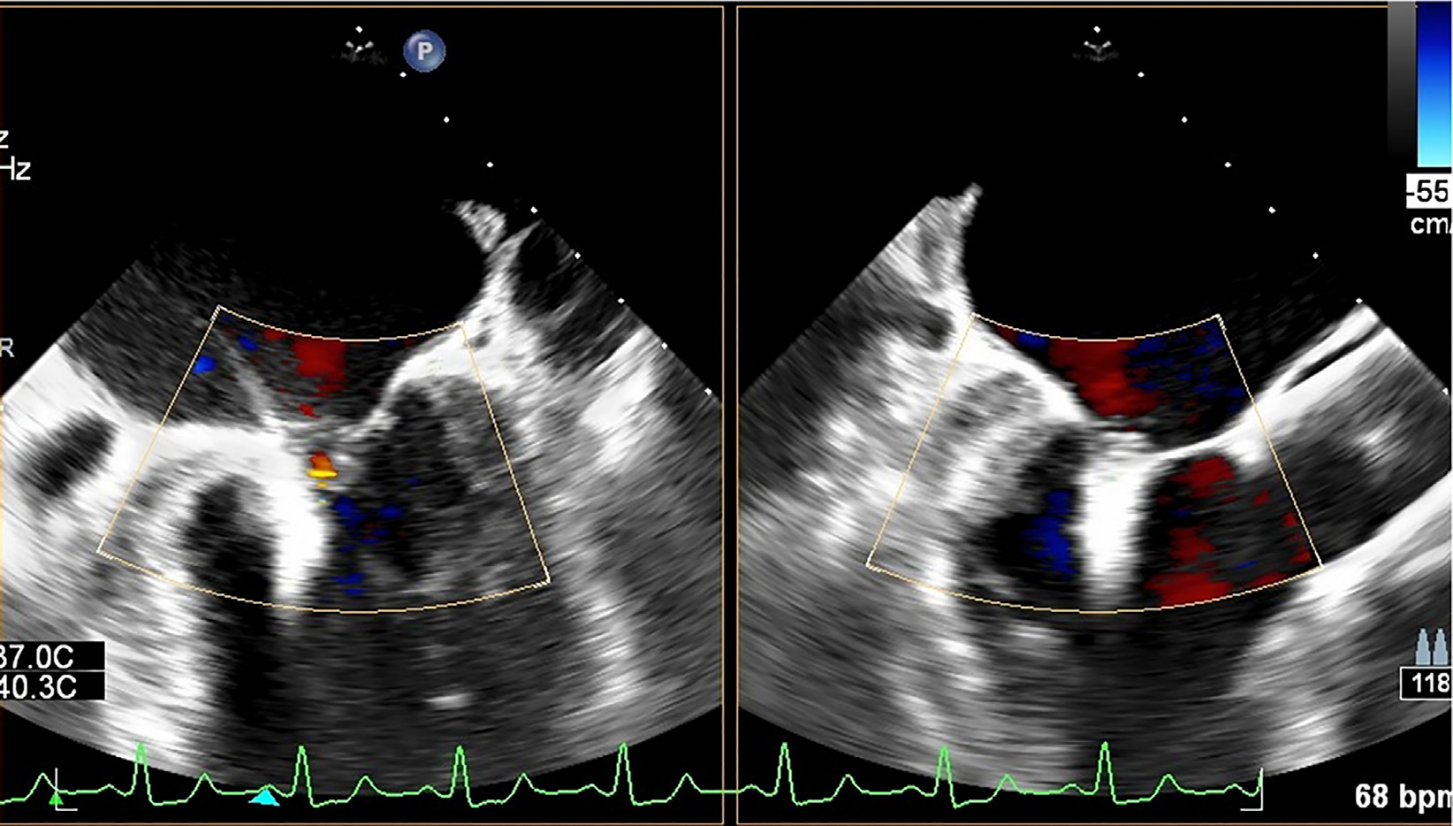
Final Result of Transcatheter Edge-to-Edge Repair by Transesophageal Echocardiography The first clip sized by 06 (width) and 12 (length) was implanted in posterior commissural leaflet P3. And another clip sized by 06 (width) and 12 (length) was implanted in A3 to P3 from shoulder to shoulder. Transesophageal echocardiography showed mitral regurgitation reduced from 4+ to trace. bpm = beats/min.
